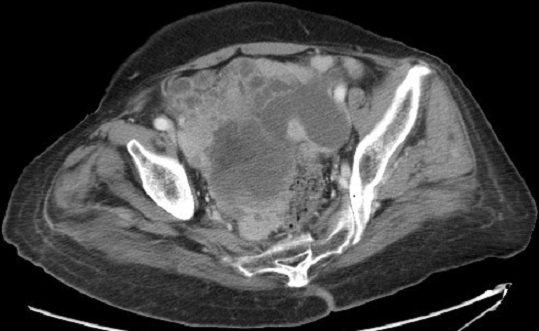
CT scan cut before chemotherapy showing peritoneal implants, right adnexal masse of 6.8 × 9.9 cm
